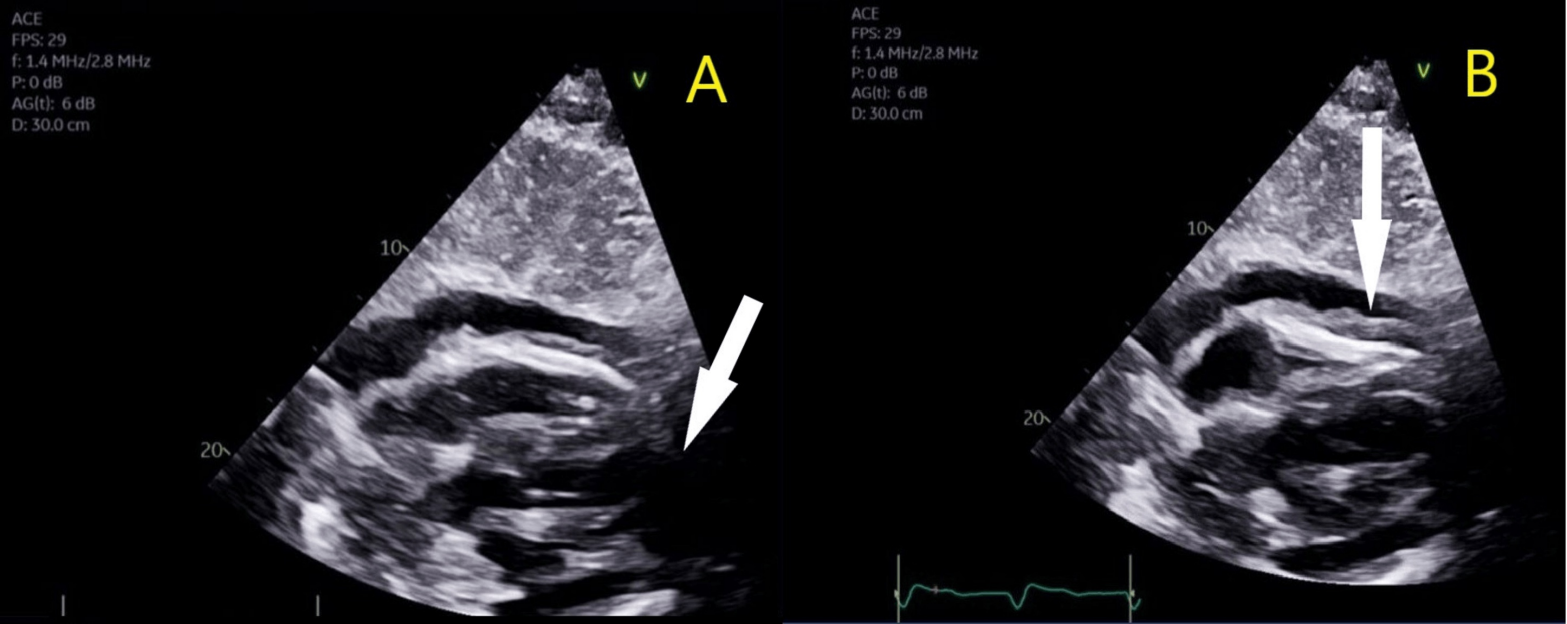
Bedside POCUS showing a large pericardial effusion on apical/subcostal views (panel A, white arrow) and RV collapse during diastole (panel B, white arrow). POCUS, point-of-care ultrasound; RV, right ventricle
